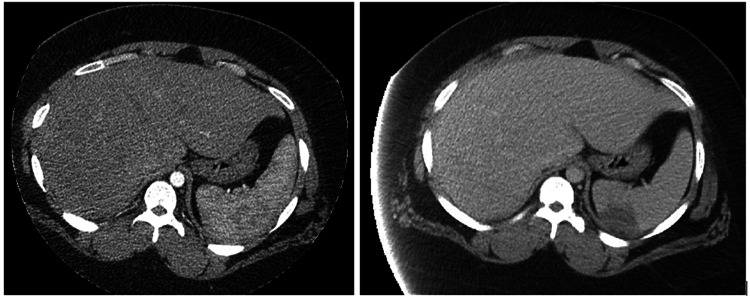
Abdominal CT showing a new 4.3-cm splenic infarct compared to the initial chest CT lower films of the previous day
